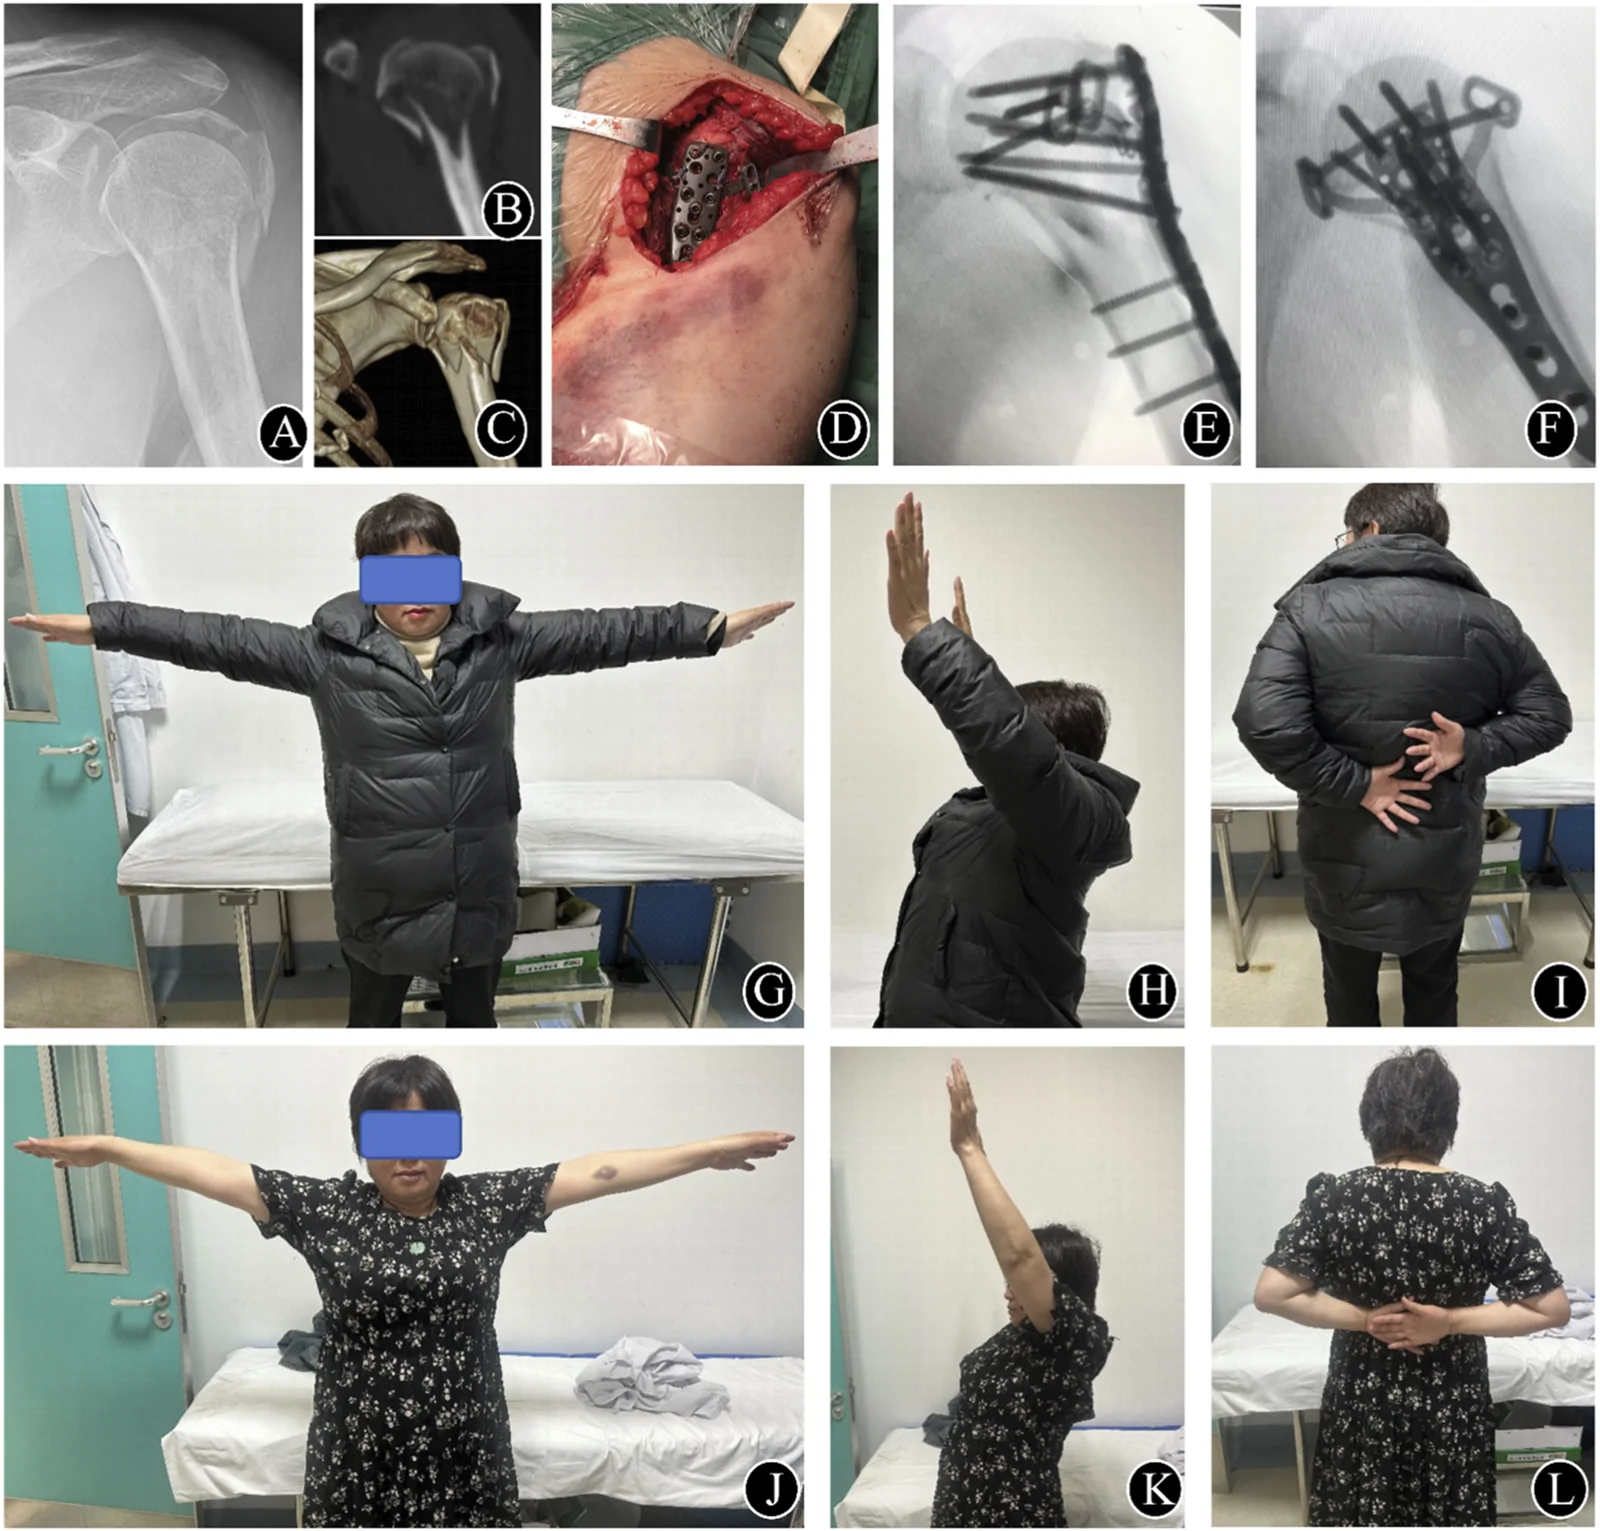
Case 1: dual-wing plate (48-year-old woman, Neer four-part fracture). (A–C) Preoperative radiographs and CT; (D–F) intraoperative fluoroscopy; (G–I) 3-month follow-up; (J–L) 6-month follow-up with maintained reduction and full functional recovery.
